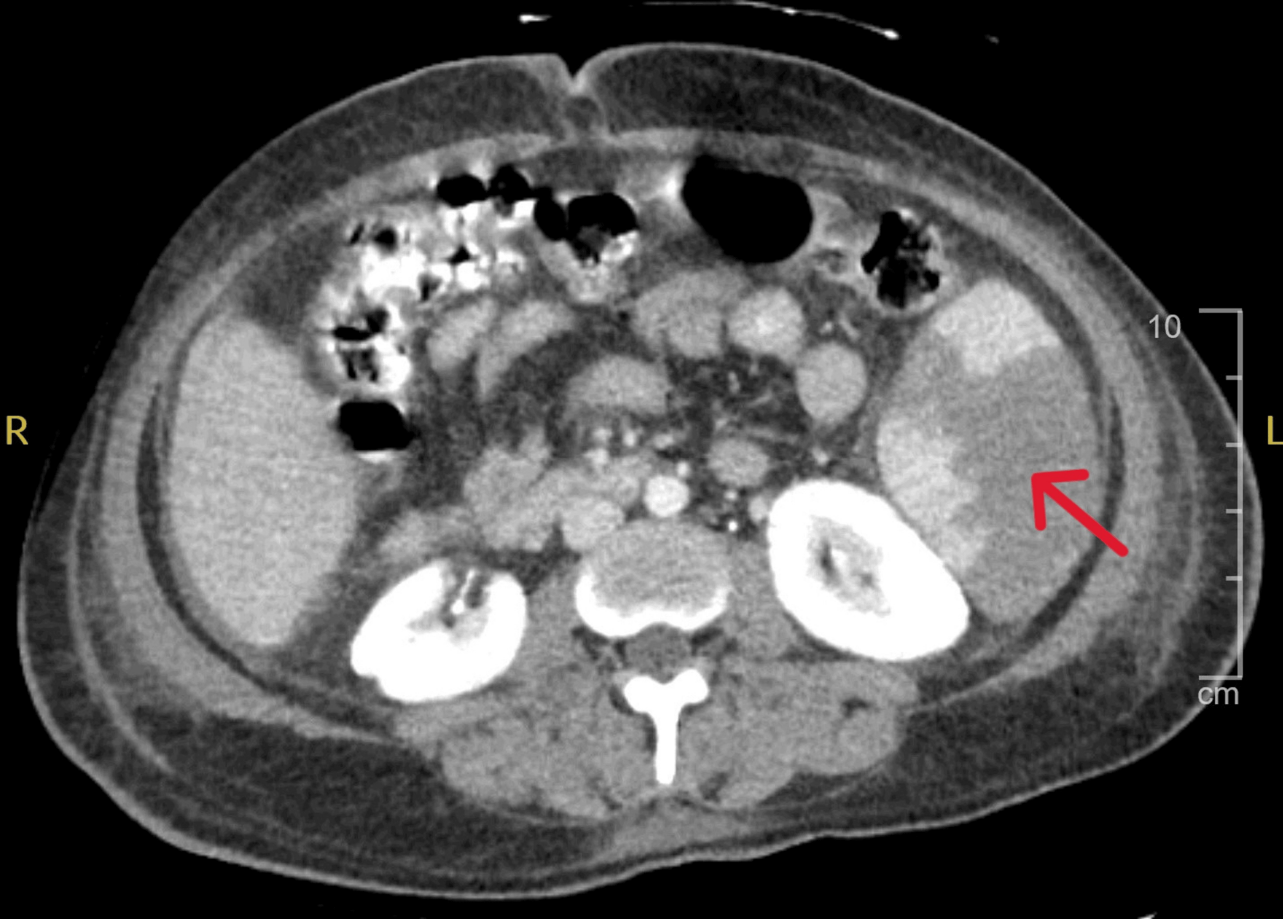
CT of abdomen and pelvis showing splenomegaly with a splenic infarct in the inferior pole. CT: computed tomography
